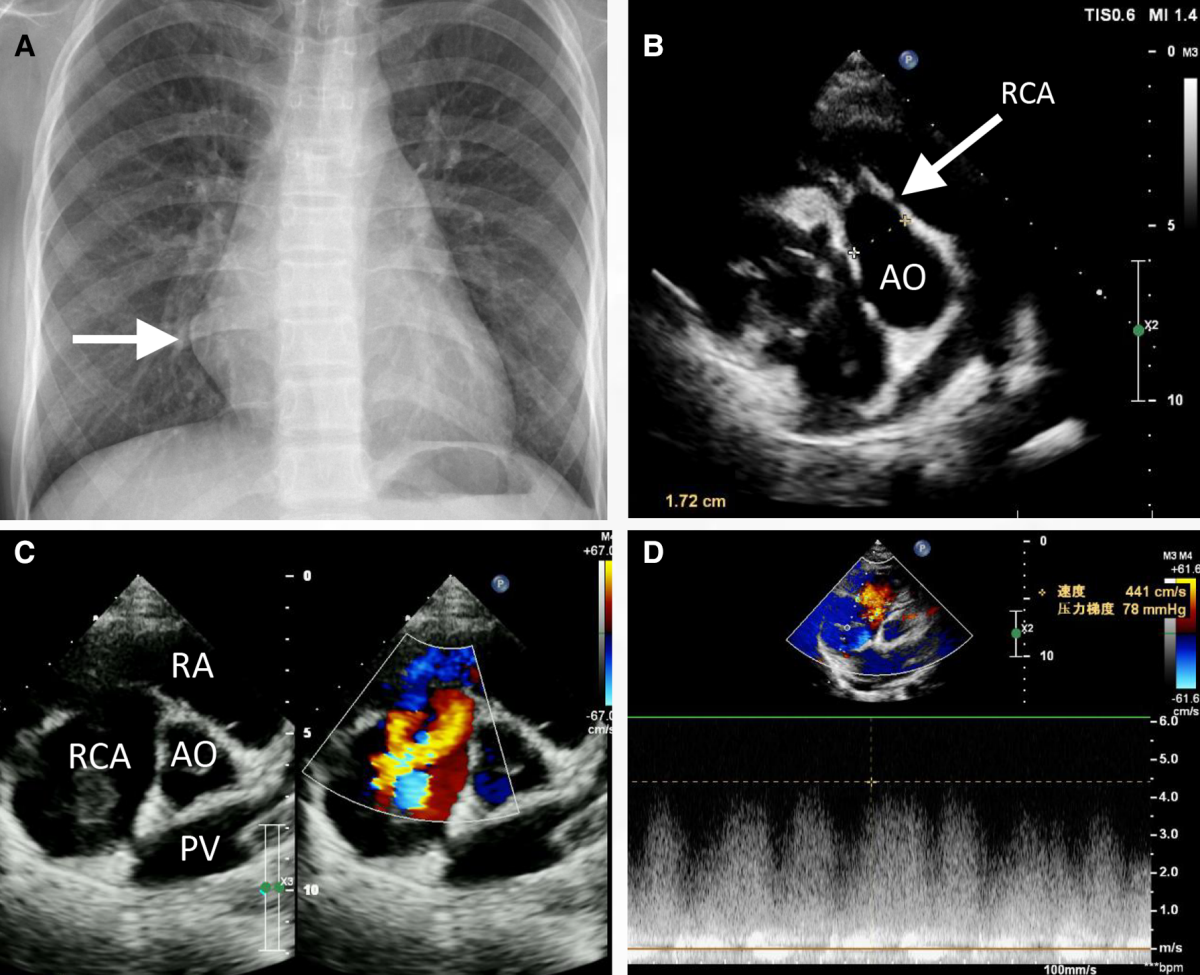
Preoperative chest radiography (A) and transthoracic echocardiogram (B, C) revealed the partially enlarged heart shadow (A, arrow), right coronary artery enlargement (B, arrow), pseudoaneurysm (C), and right coronary artery to right atrium fistula (C, D). AO, ascending aorta; PV, pulmonary vein; RA, right atrium; RCA, right coronary artery.
